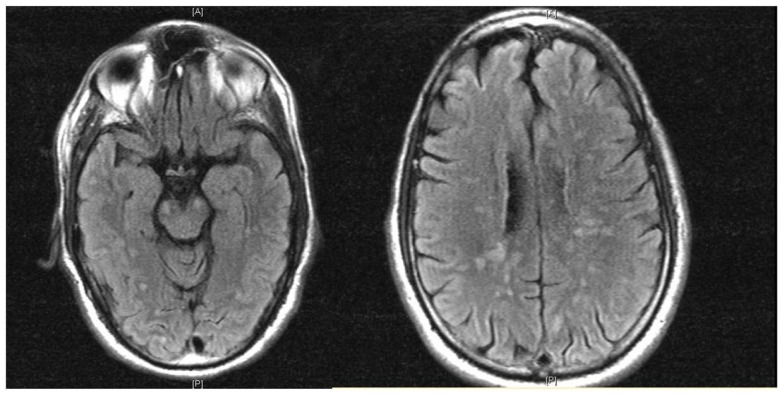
MRI (FLAIR) shows multiple rounded lesions throughout the brain parenchyma predominantly involving the white matter of both cerebral hemispheres, basal ganglia and midbrain.
